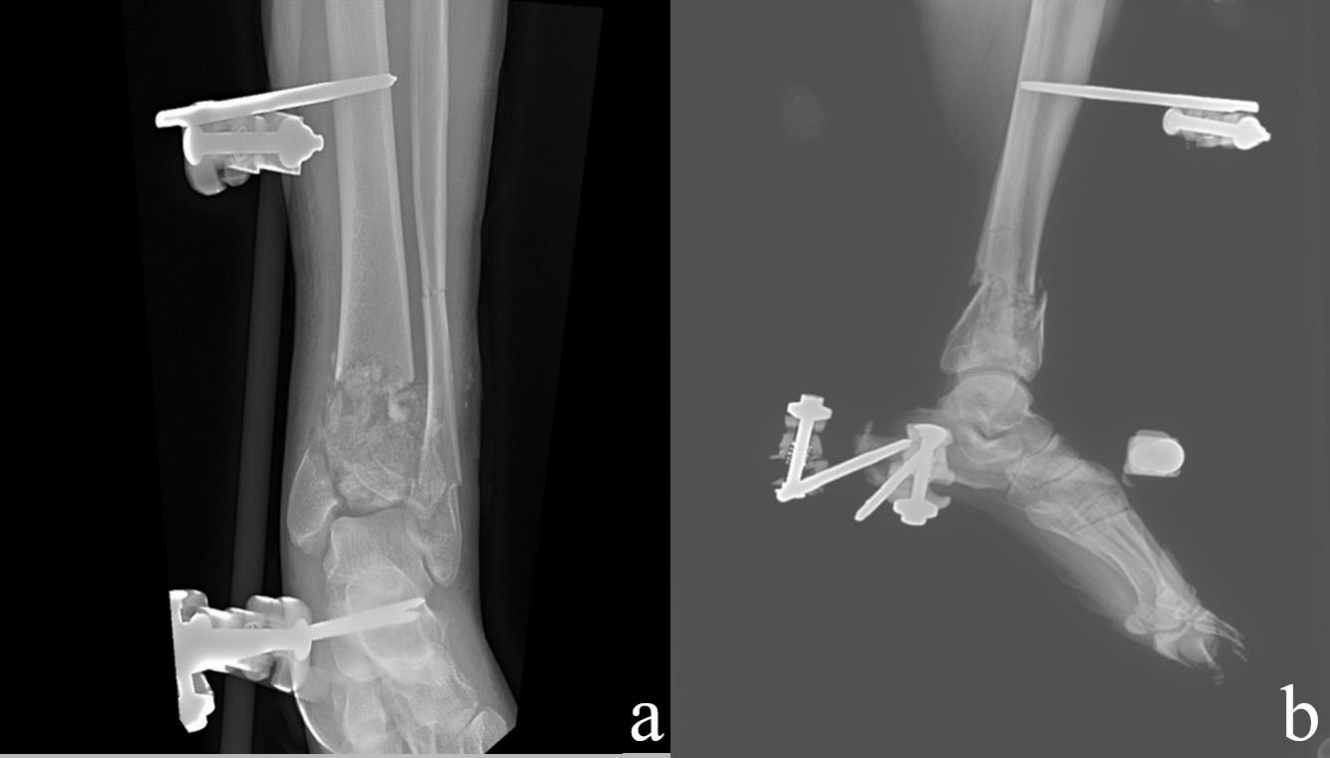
Anteroposterior (a) and lateral (b) x-ray images after external fixation (first step of two stage surgery).
